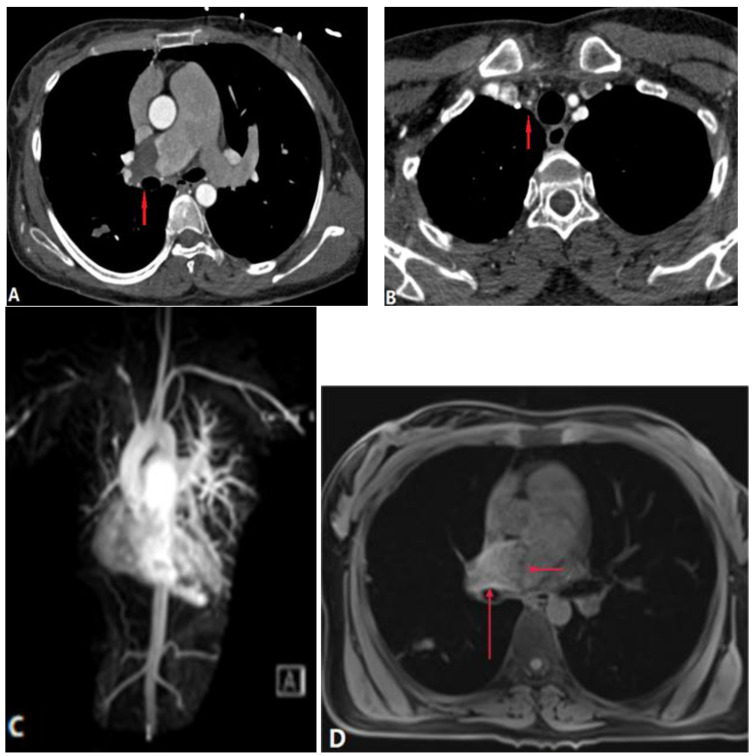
Vasculitis-related pulmonary artery thrombosis. Takayasu arteritis in a 33-year-old woman with dizziness and chest compress. (A): Contrast-enhanced axial CT demonstrates a filling defect and occlusion of the right pulmonary artery wall (arrow), and the main pulmonary is dilated. (B): Axial CT image shows the thickening walls of the right subclavian artery and right common carotid artery, and the lumen is narrow (arrow). (C): Coronal MIP contrast-enhanced MRA image shows that the right brachiocephalic artery, right subclavian artery, and right common carotid artery are stenosed or occluded; the right pulmonary artery and distal segment are stenosed. (D): Axial MR T1 image shows mild thickening of the right pulmonary artery (long arrow) and iso-intensity thrombosis (short arrow). MIP—maximum-intensity projection; MRA—magnetic resonance angiography.
